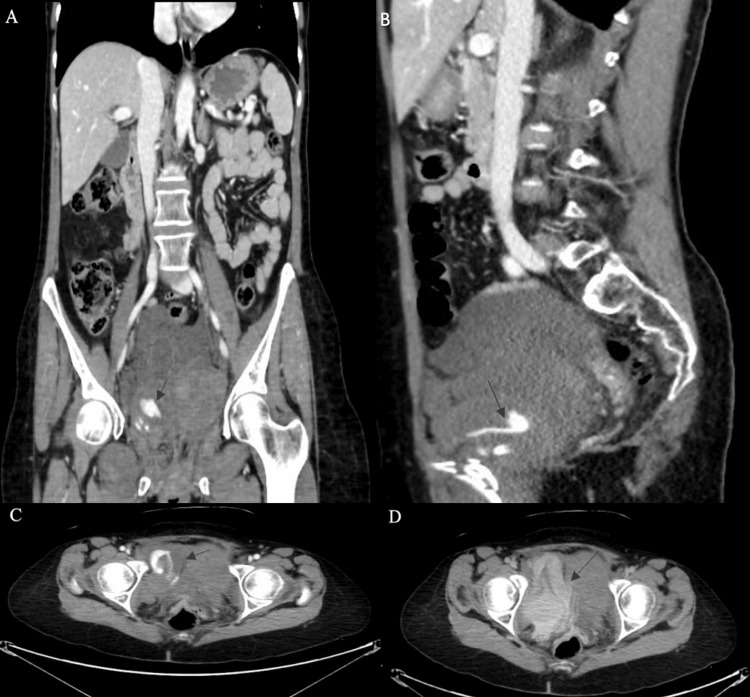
CT of the abdomen and pelvis with contrast showing a substantial pelvic hematoma in the coronal and sagittal views (A,B), while in the transverse sections (C,D), the presence of contrast extravasation within the hematoma unequivocally indicates an ongoing active hemorrhage (arrows) CT: computed tomography
